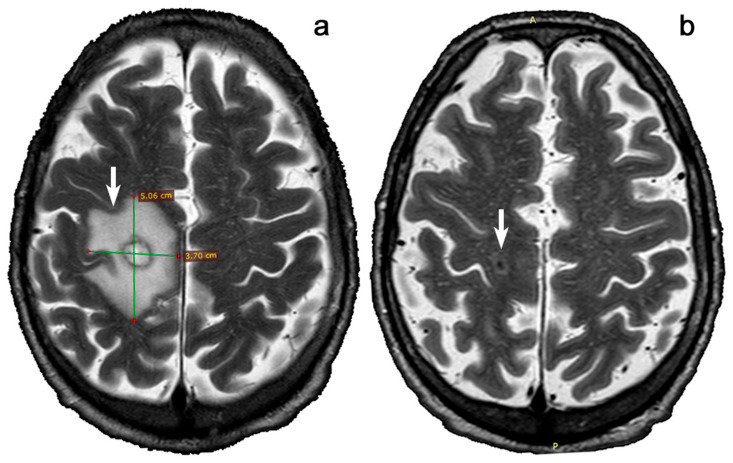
Brain MRI scan showing neuro-toxoplasmosis before and after treatment. (a). At baseline, a 5.3 × 3.7 cm nodular ring-enhancing lesion is evident in the right hemisphere (arrow); (b). The Toxo-g abscess, present at baseline in the right hemisphere, appears significantly reduced after a 6 weeks treatment course with trimethoprim/sulfamethoxazole (arrow).
